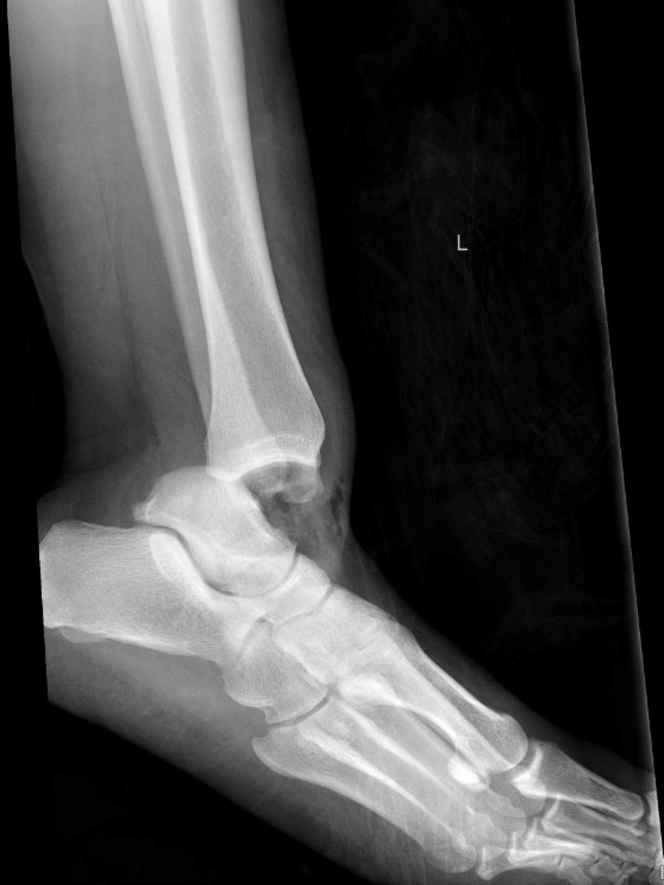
Initial ankle radiograph demonstrating locules of gas in soft tissue.
